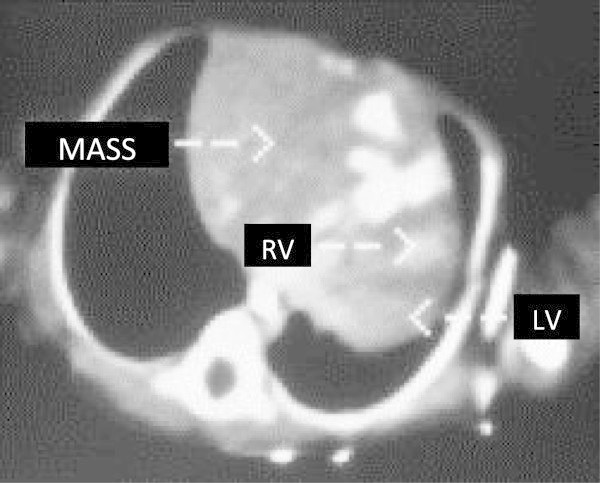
CT scan of the chest demonstrates the mass (identified) to the right of the heart. RV = Right Ventricle, LV = Left Ventricle.
